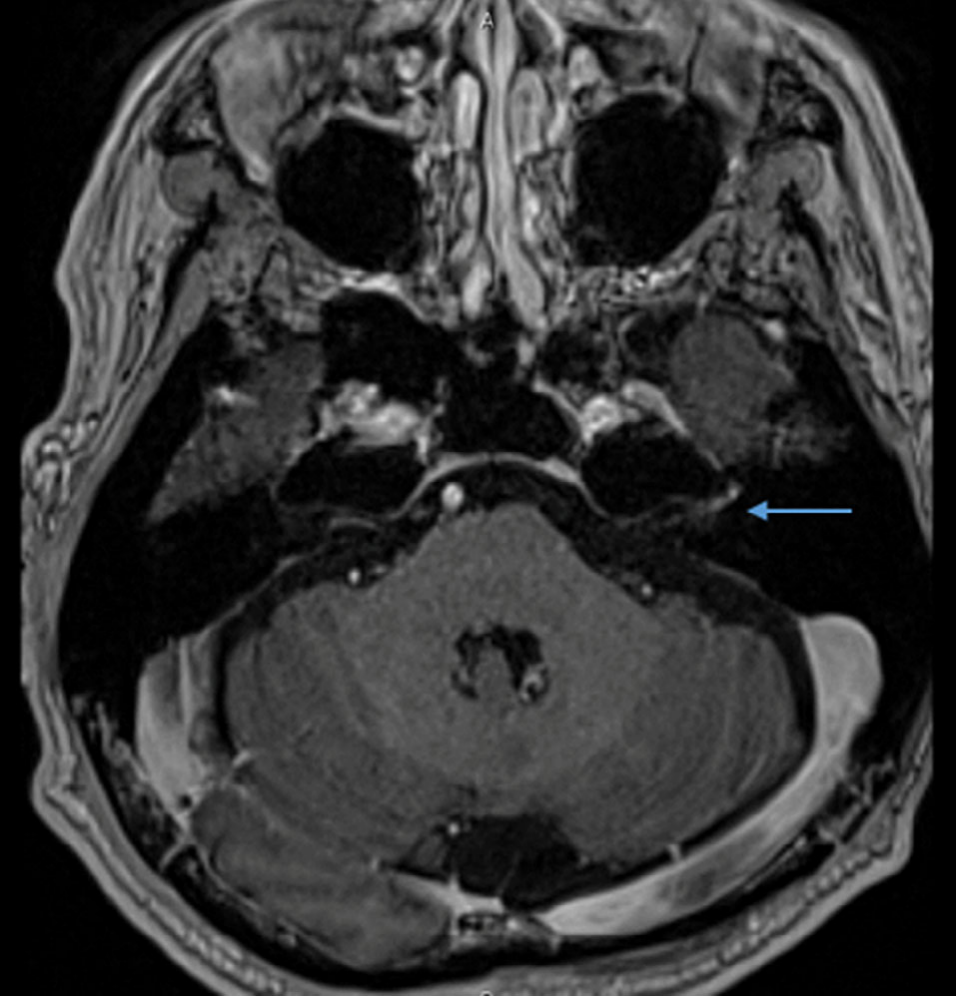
T1 weighted MRI image with contrast medium. The blue arrow shows the enhancement of the left facial nerve.
